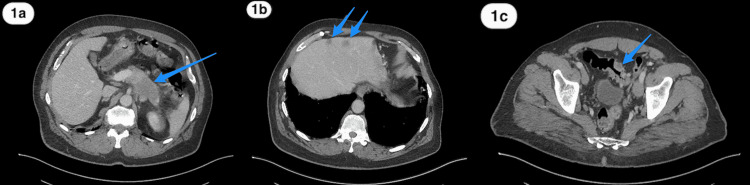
Abdomen CT scan (a) A contrast-enhanced abdomen CT scan axial image showing an ill-defined non-enhancing low-density solid mass in the pancreatic body and tail (blue arrow). (b) A contrast-enhanced abdomen CT scan axial image showing liver metastases (blue arrows). (c) A contrast-enhanced pelvis CT scan axial image showing focal non-uniform wall thickening of the sigmoid colon (blue arrow). CT, computed tomography.
